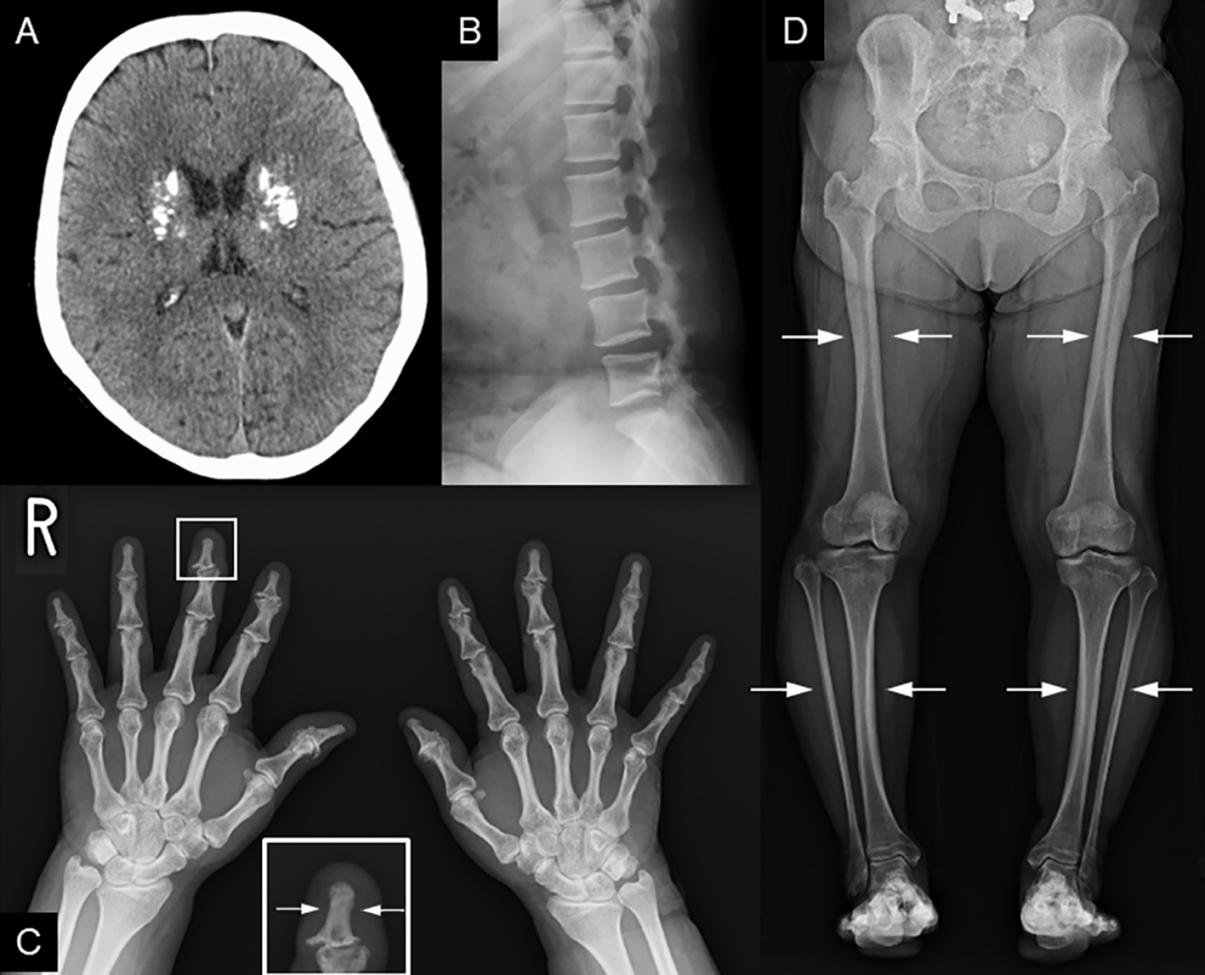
Radiological findings. (A) Head CT shows coarse calcification of the capsule. (B) Preoperative image of lumbar spondylolisthesis at age 48. Disc space irregularity is seen between L4 and L5. (C, D) Cortical thickening of the bones, particularly of the (C) fingers and lower leg bones (i.e, femur, tibia, and fibula). (D) Medullary stenosis of bones is also observed.
